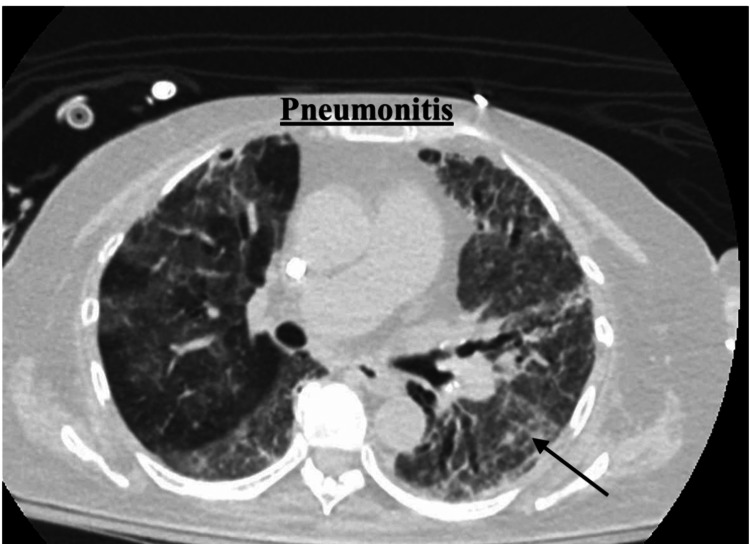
CT scan imaging: pneumonitis The pulmonary manifestation of anti-GBM disease is characterized by immune-mediated pneumonitis secondary to autoantibody-driven injury of alveolar basement membranes. These are seen as ground glass opacities in our patient and secondary to an ongoing inflammatory process, suspicious for an autoimmune cause. CT, computed tomography
